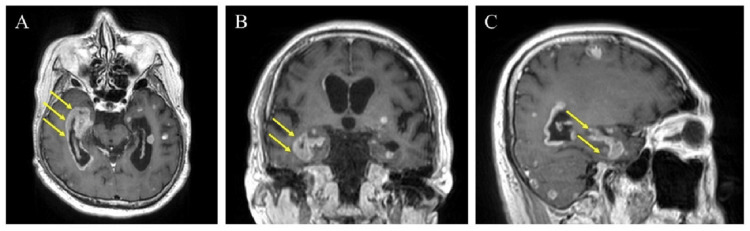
MRI images of meningeal carcinomatosis in the perihippocampus following hippocampal avoidance prophylactic cranial irradiation. The images show axial (A), coronal (B), and sagittal (C) contrast-enhanced T1-weighted images. Meningeal carcinomatosis is visible as solid enhancing masses in the perihippocampus (arrows in A-C).
